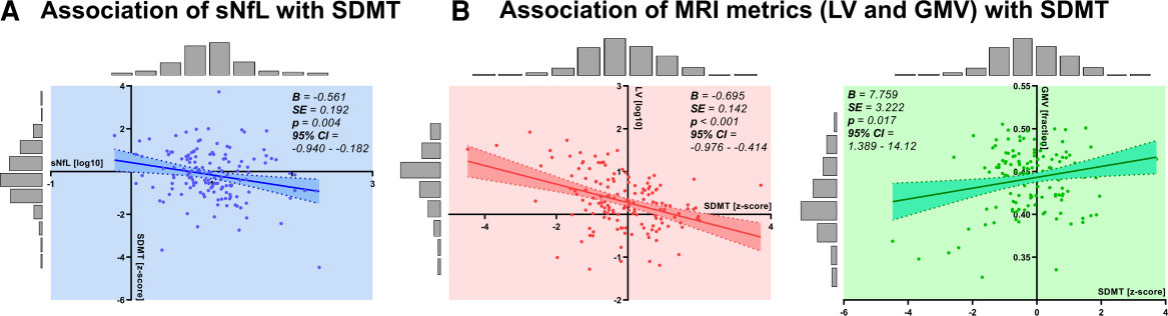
Relationship between individual markers and SDMT performance. (A) Associations of sNfL levels (log-transformed) with SDMT (z-scores). *N* = 149, *P* = 0.004; 95% CI = −0.940 to −0.182. (B) Association of MRI metrics [LV (log10-transformed) and GMV (fraction)] with SDMT (*z*-scores) each with the corresponding 95% confidence intervals. *N* = 152, LV: *P* < 0.001, 95% CI = −0.976 to −0.414; GMV: *P* = 0.017, 95% CI = 1.389–14.128. Histograms represent the data distribution of the cohorts. The *P*-value was adjusted for age, sex, EDSS, disease duration and DMT. B = Regression coefficient derived from linear regression; SE = Standard error derived from linear regression.
